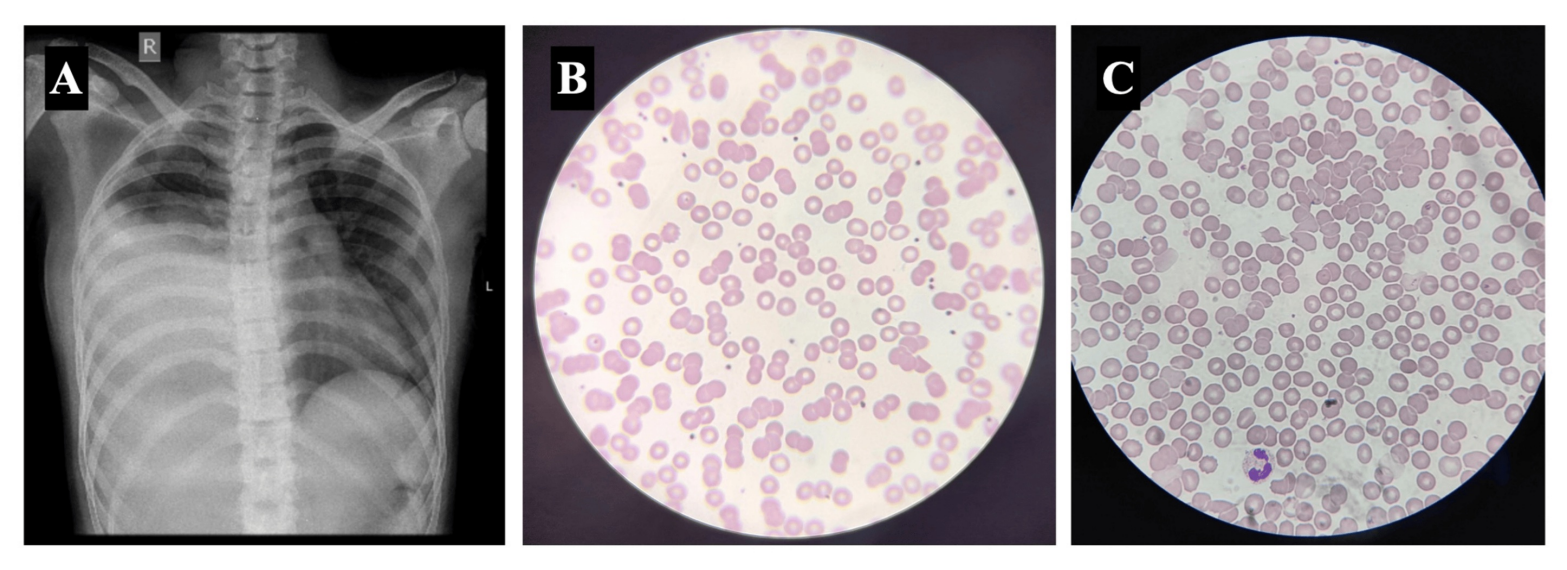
Chest radiograph and peripheral blood smear findings throughout the clinical course. (A) Chest radiograph obtained during the second week of illness showing progressively increasing opacity in the right mid and lower zones. (B) Peripheral blood smear during admission showing normocytic normochromic anemia. (C) Peripheral blood smear during the second week of illness showing clumping of RBC, suggestive of hemolysis. RBC: red blood cell
